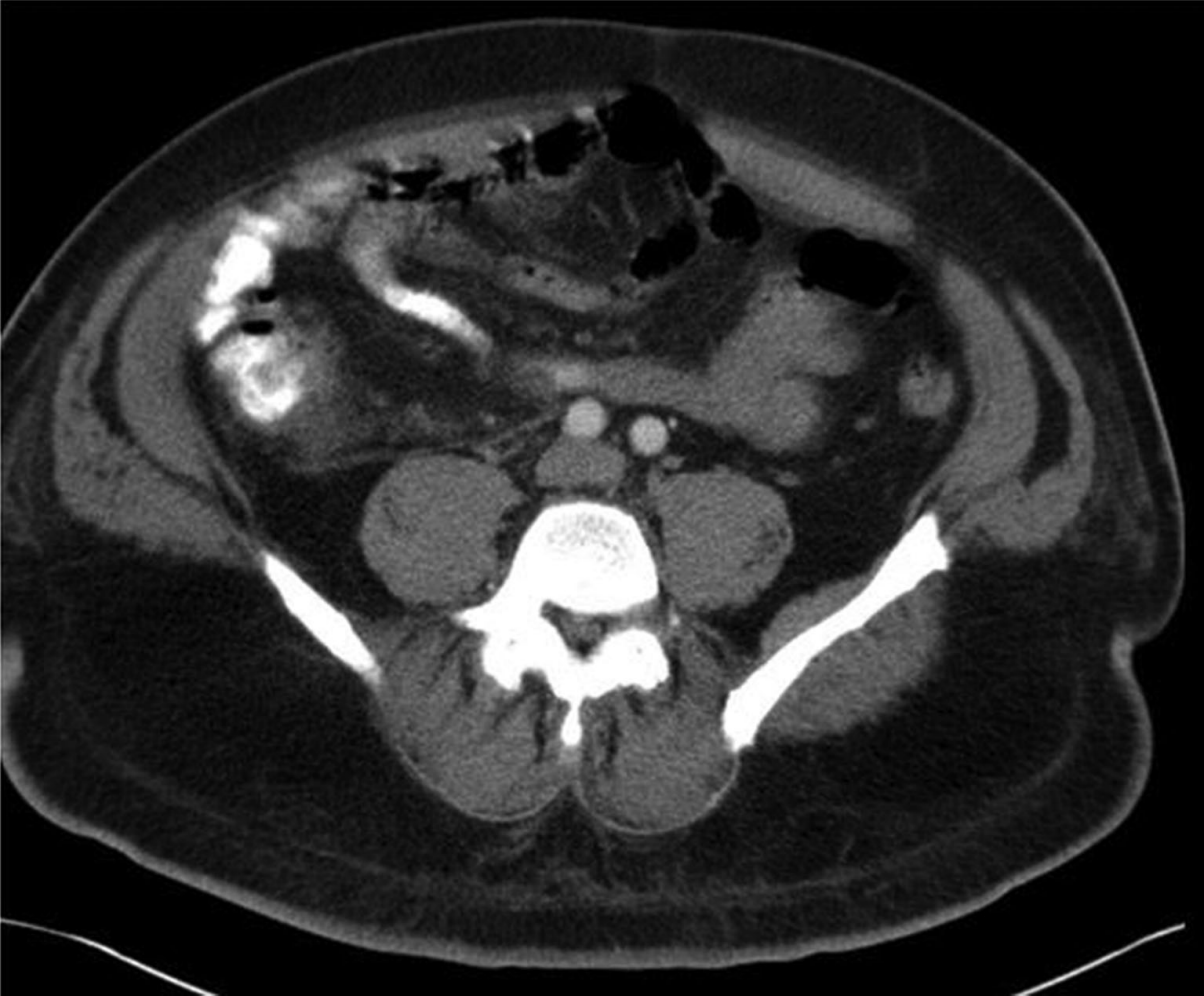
Contrast-enhanced CT of the abdomen on hospital day 7 showing resolution of the ileocecal mass.
